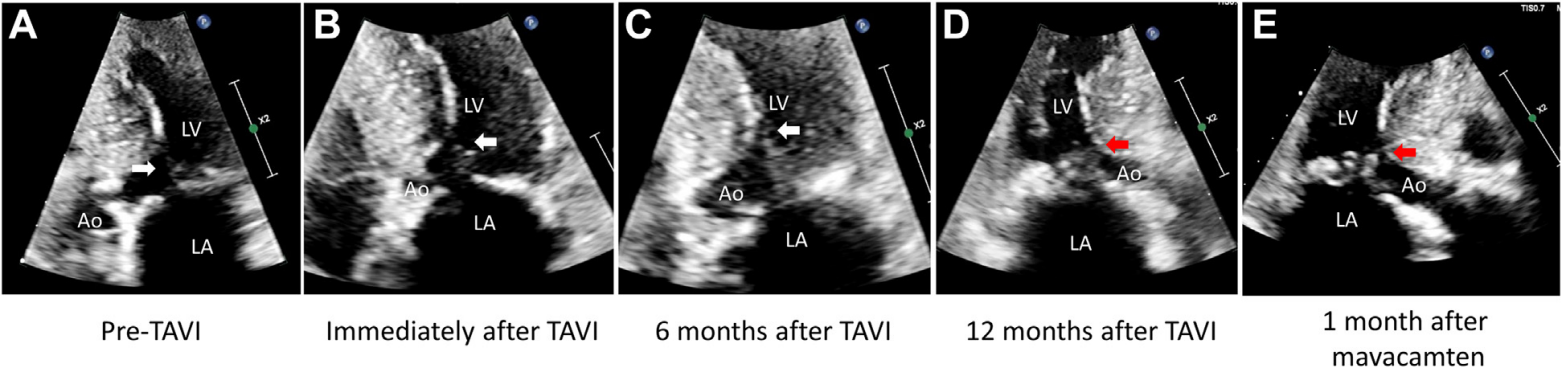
Serial Echocardiographic Images of SAM Before TAVI, SAM was absent (A, white arrow), and immediately after successful TAVI, prominent SAM appeared (B, white arrow), which showed progressive septal contact (C and D, white and red arrows). Mavacamten treatment resulted in less septal contact of SAM (E, red arrow). Abbreviations as in Figure 1.
